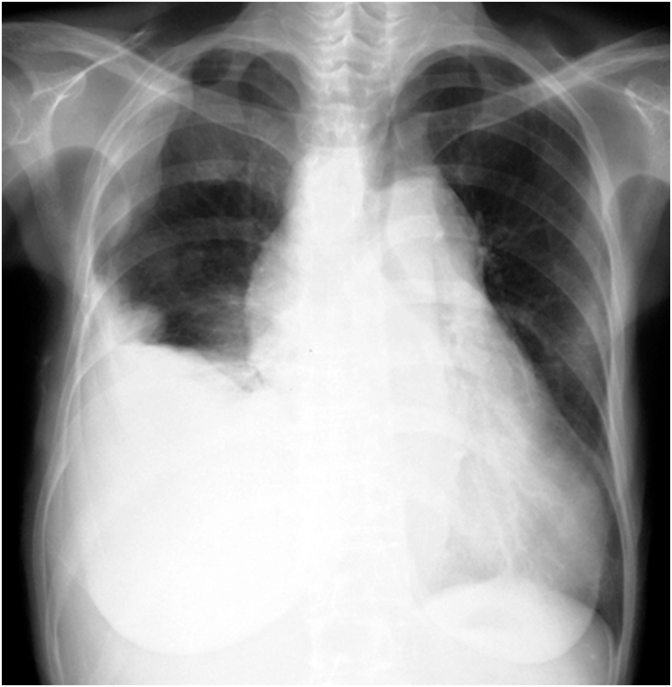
Chest radiographs taken on admission to Toyama Rosai Hospital (May 2010) The inside of the right thorax was mostly occupied by multiple masses, and the mediastinum is displaced to the left.
